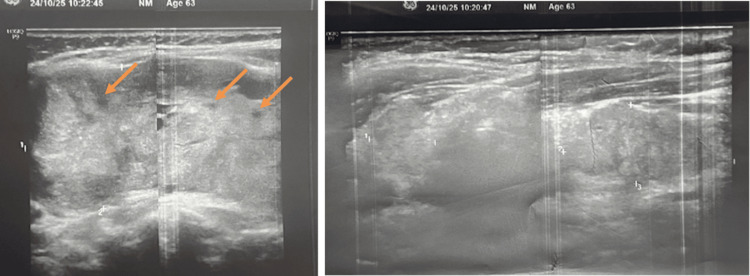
Thyroid ultrasonography demonstrating suspicion for malignancy: multiple small nodules indicated with the arrows.
